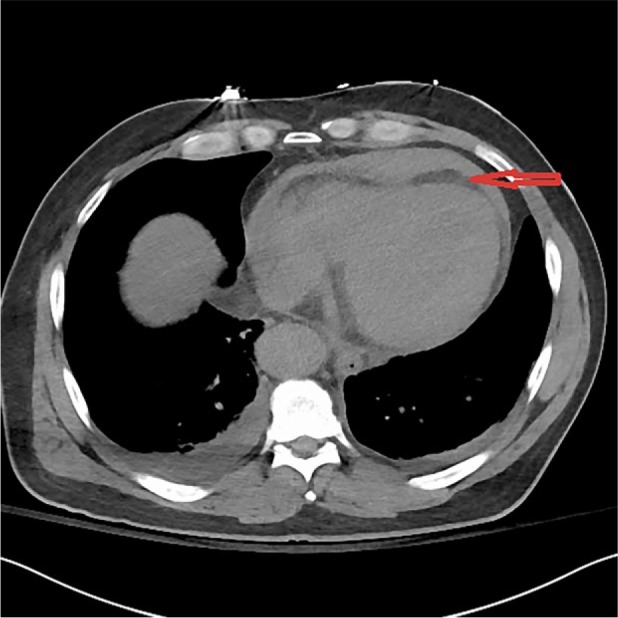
Arrow showing pericardial effusion on non-contrast CT. No dissection flap is seen.
